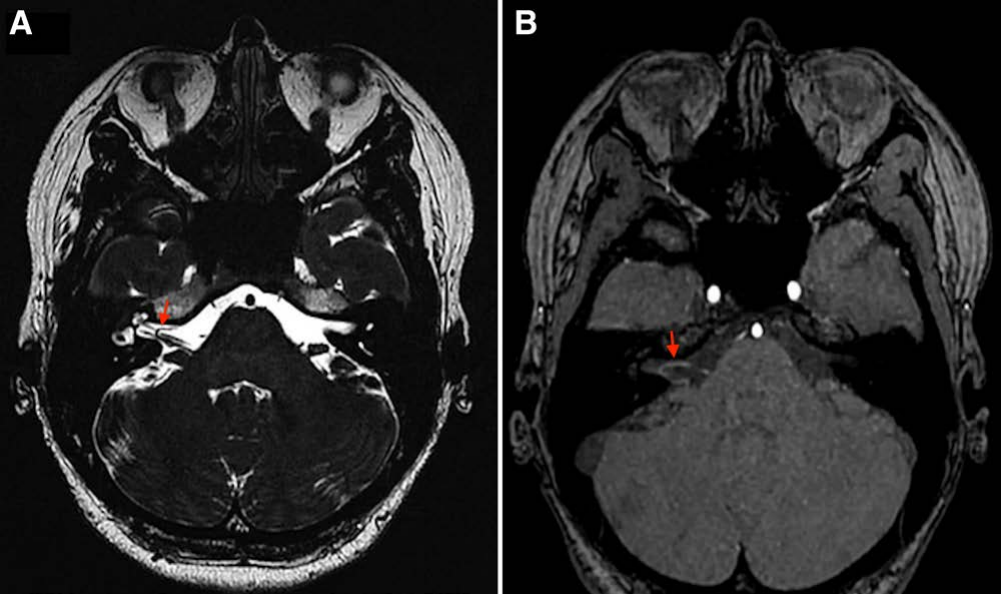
(A and B) Are 3D-TOF MRI showed that the right CN VII penetrated into the IAC and was closely related to the AICA loops (red arrows).
